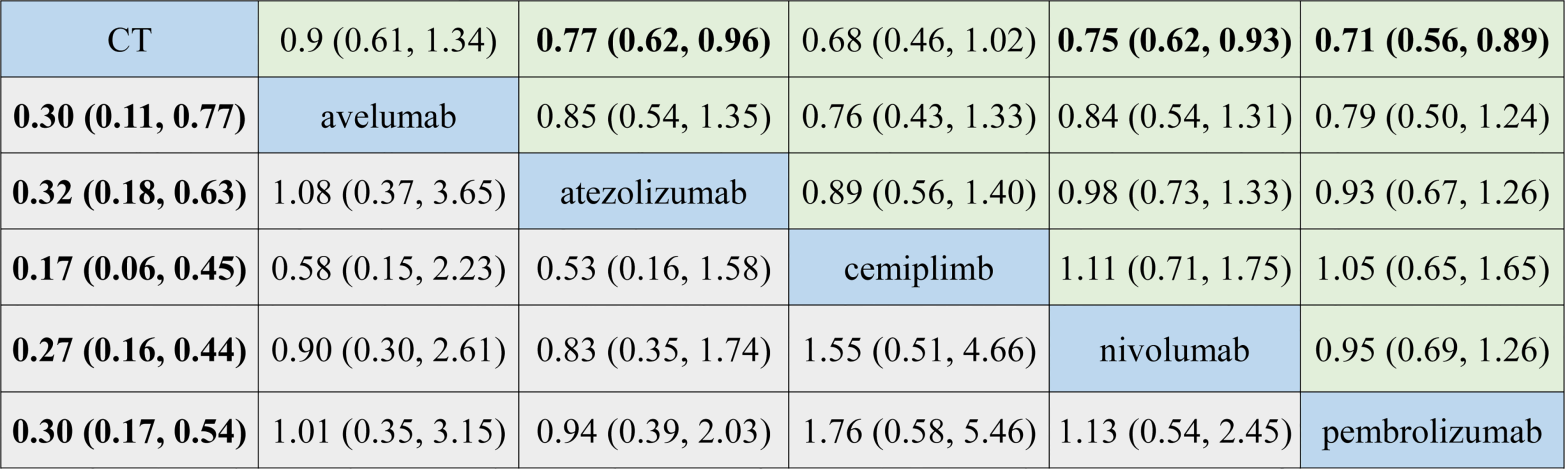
Summary for target outcomes including overall survival (OS) and treatment-related adverse events (TRAEs). The upper right section presented results for OS, and the left bottom section showed the results for TRAEs. The number in each cell represented the result that the treatment in the upper left cell divides that treatment in the lower right cell. Bold number indicates statistical significance. CT, chemotherapy.
